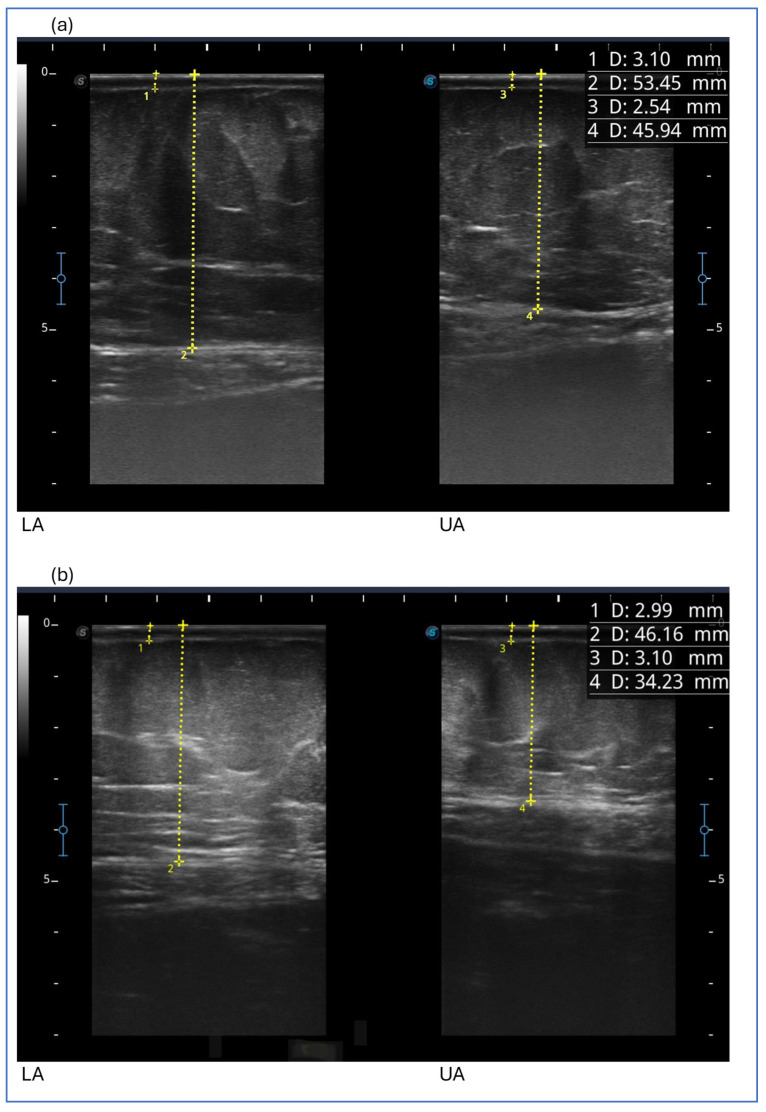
Ultrasound measurements for case report at the beginning of the study in the image above (a) and at the end of the study in the image below (b) for case report 4.
